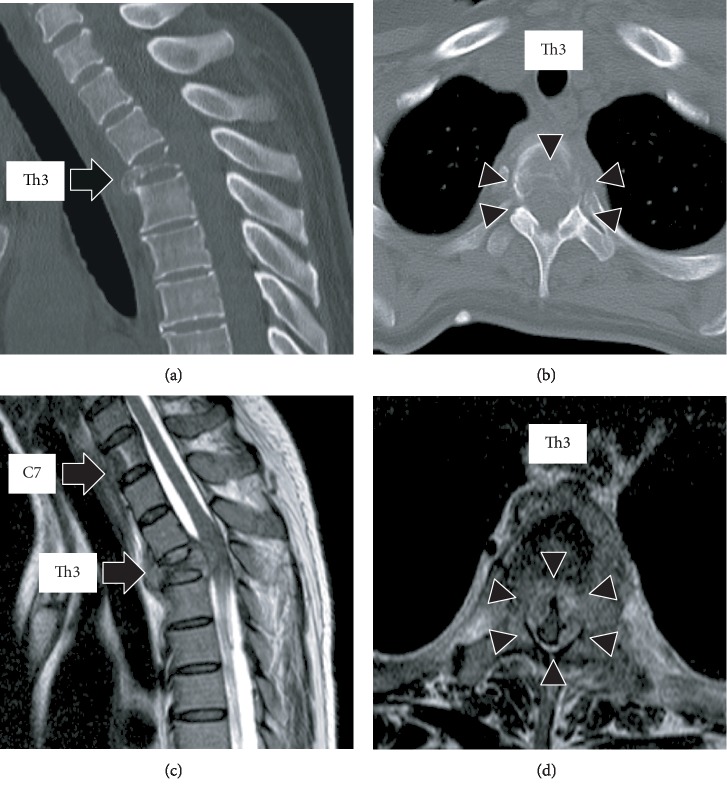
(a, b) Sagittal and axial views of the thoracic spine on computed tomography (CT) before treatment showing a collapsed Th3 vertebral body (arrow) due to the lytic lesion (arrowhead), causing kyphosis. (c, d) Sagittal and axial views of the thoracic spine on magnetic resonance imaging (MRI) showing C7 and Th3 vertebral bodies (arrow) infiltrated by the tumor. At the Th3 level, the tumor extends into the posterior vertebral body and bilateral pedicles, resulting in severe compression of the spinal cord (arrowhead).
